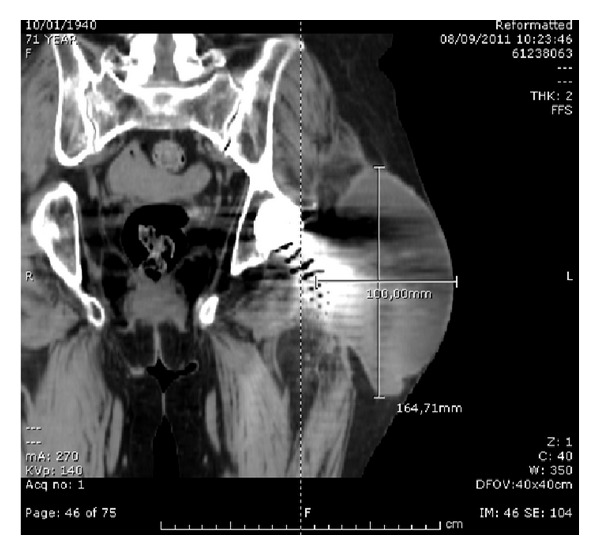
CT scan showing large septic joint fluid collection of the left hip.
